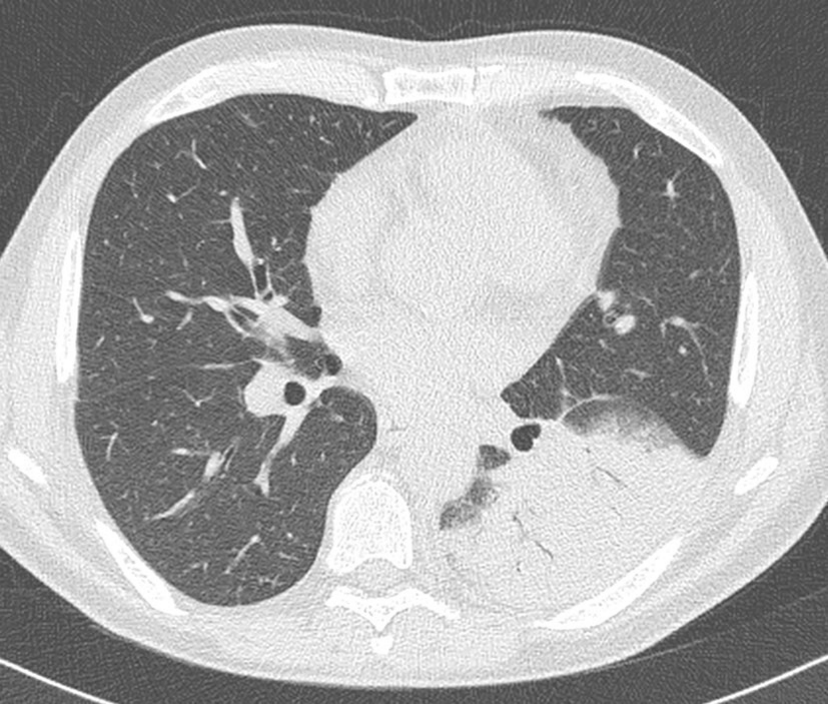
A 21-year-old man with dyspnea, cough and thoracic pain in the last two days, without fever and without history of COVID-19 exposure. CT shows a large area of consolidation with air bronchogram involving the lower lobe of the left lung suggesting bacterial lobar pneumonia. The patient, instead, was positive to the RT-PCR test
